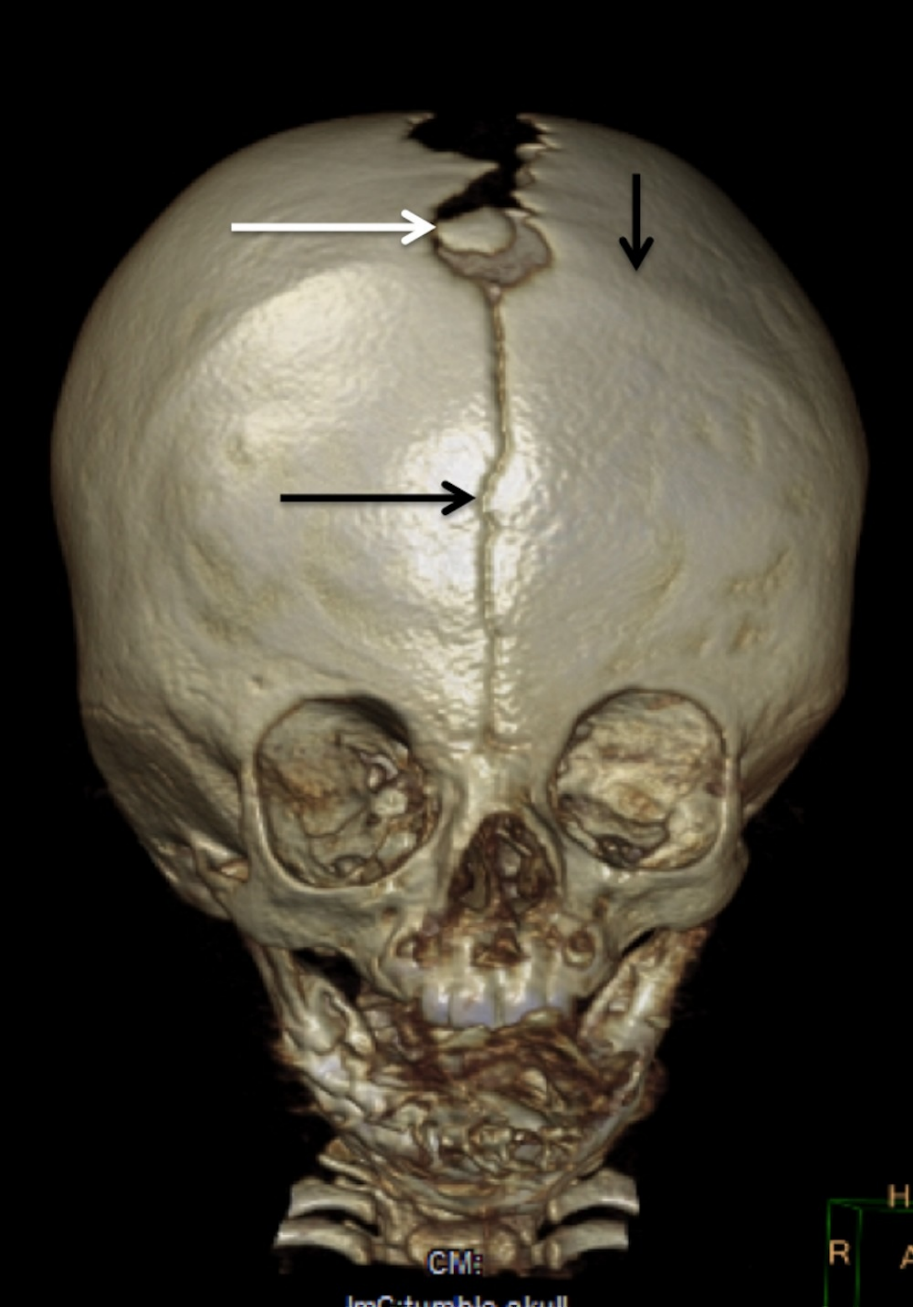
Anterior fontanellar wormian bone in a six-month-old male infant 3D computed tomography (CT) noting bilateral coronal synostosis (vertical arrow over synostosed left black coronal suture), metopic suture (lower horizontal black arrow), and anterior fontanelle wormian bone (middle white arrow).
